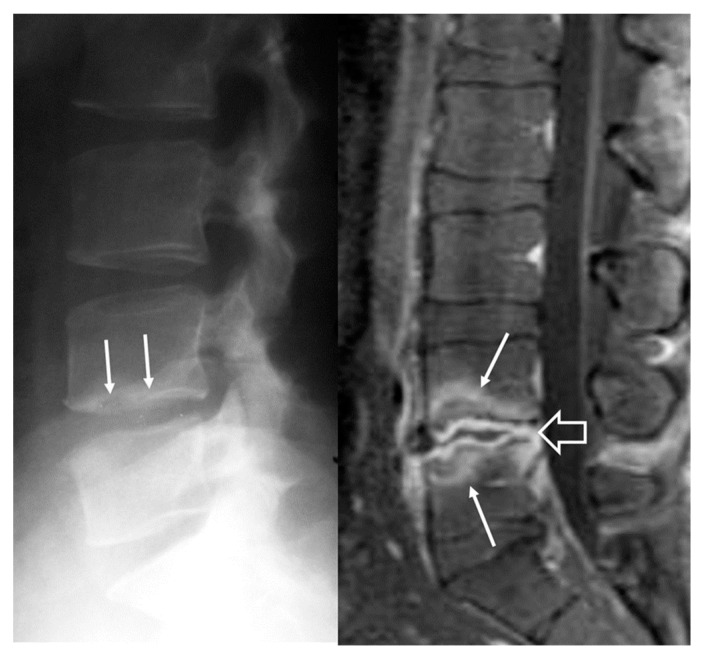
A 34-year-old man with *Brucella* spondylodiscitis. The initial lateral radiograph (left) shows a cortical disruption at the inferior epiphyseal plate of L4 vertebral body (arrows). The sagittal fat suppressed contrast enhanced T1-w MR image (right) shows septic discitis (open arrow) and bone barrow edema on both L4 and L5 vertebral bodies (arrows), suggesting spondylitis.
